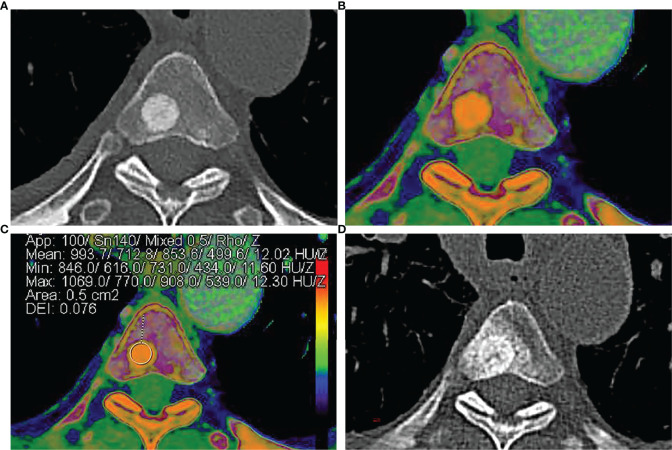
A 58-year-old man was diagnosed with lung adenocarcinoma. (A) Axial contrast-enhanced CT image shows an intra-osseous focal hyperdense lesion with a clear margin in the 4th thoracic vertebral body. (B, C) Rho/Z on DECT shows the placement of ROI over an intra-osseous focal hyperdense lesion. Values of ROI: Z = 12.02, Rho = 499.6, DEI = 0.076, rCT = 853.6. (D) Size and density of the intra-osseous focal hyperdense lesion were both increased in 1-year follow-up CT images, and the lesion was diagnosed as OBM.
